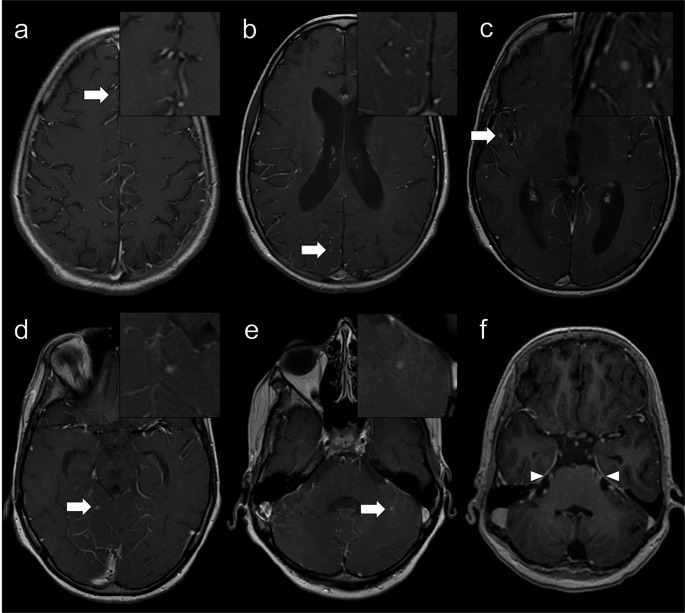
Chronic granulomatous disease. A 16-year-old male patient with meningitis caused by *Granulibacter bethesdensis* and *Rhizobium radiobacter*. The patient has a history of lymphadenitis, pneumonia, and sepsis without an identified causative agent. Gadolinium-enhanced axial T1-weighted images reveal multiple nodular enhancing lesions in the cerebral and cerebellar subcortical regions (arrows), without low signal on ADC maps (not shown), likely representing microabscesses. There is also leptomeningeal enhancement, most pronounced in the posterior fossa involving the medulla and trigeminal nerves (arrowheads), and mild supratentorial hydrocephalus
